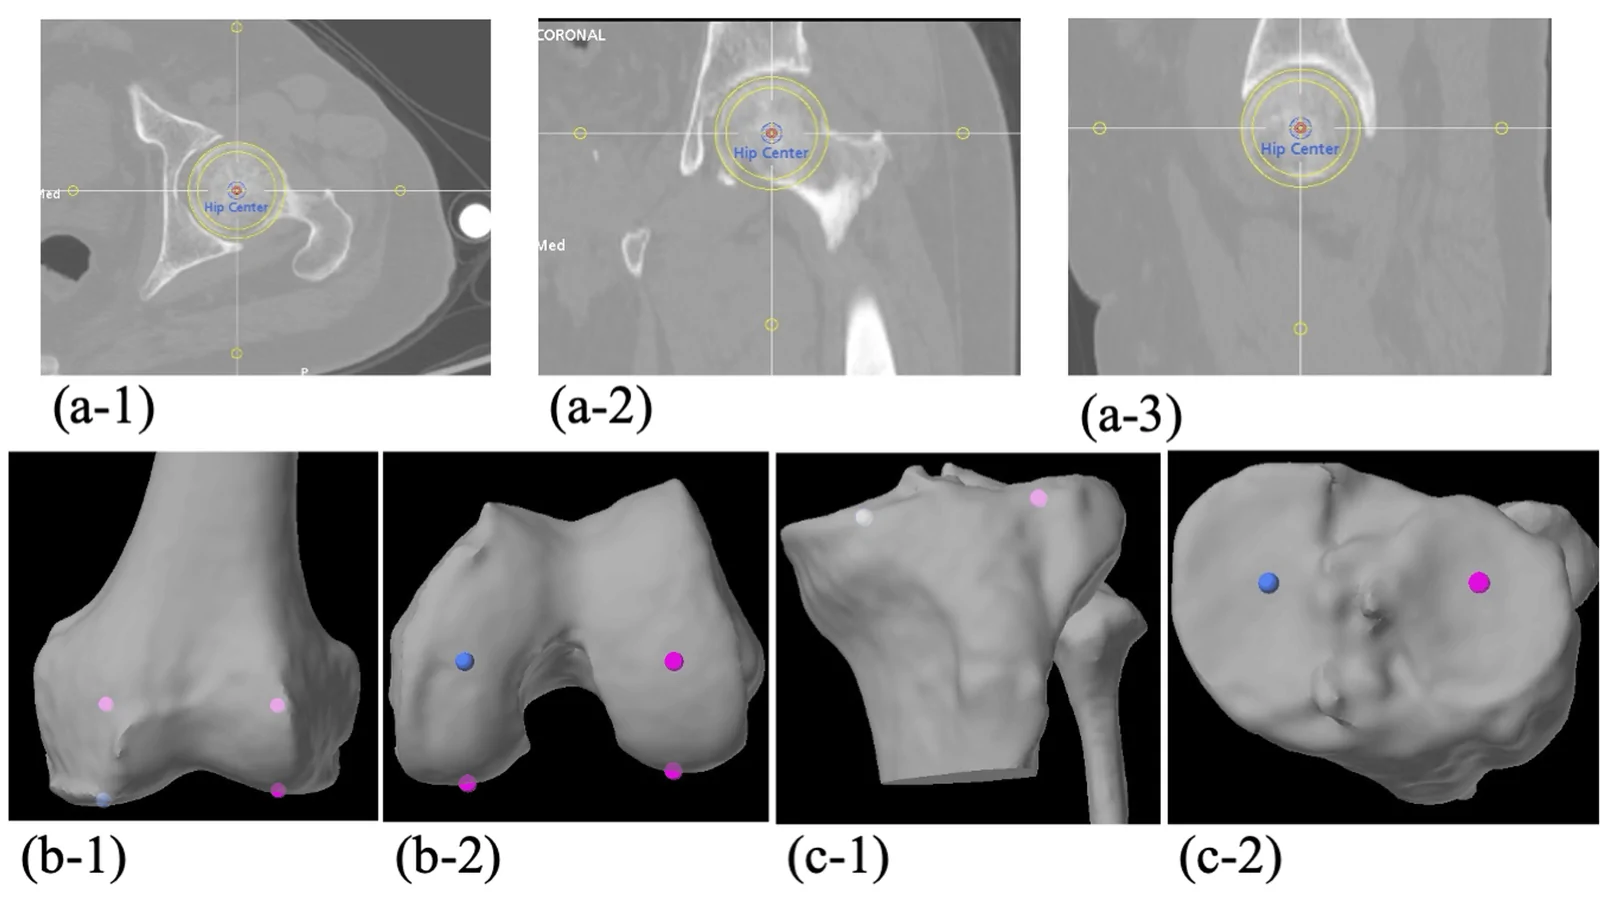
Measurement landmarks on CT images (a-1) Center of the femoral head on the axial view. (a-2) Femoral center on the coronal view. (a-3) Femoral center on the sagittal view. (b-1, 2) Landmarks of the femoral condyles. (c-1,2) Landmarks of the proximal tibial joint surface CT: computed tomography
